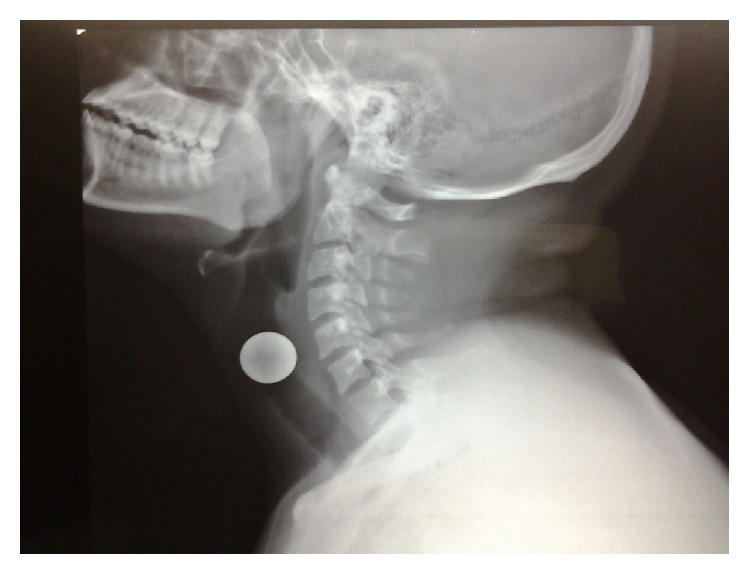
Lateral X-ray: lateral view showing rounded opacity in sagittal plane.
